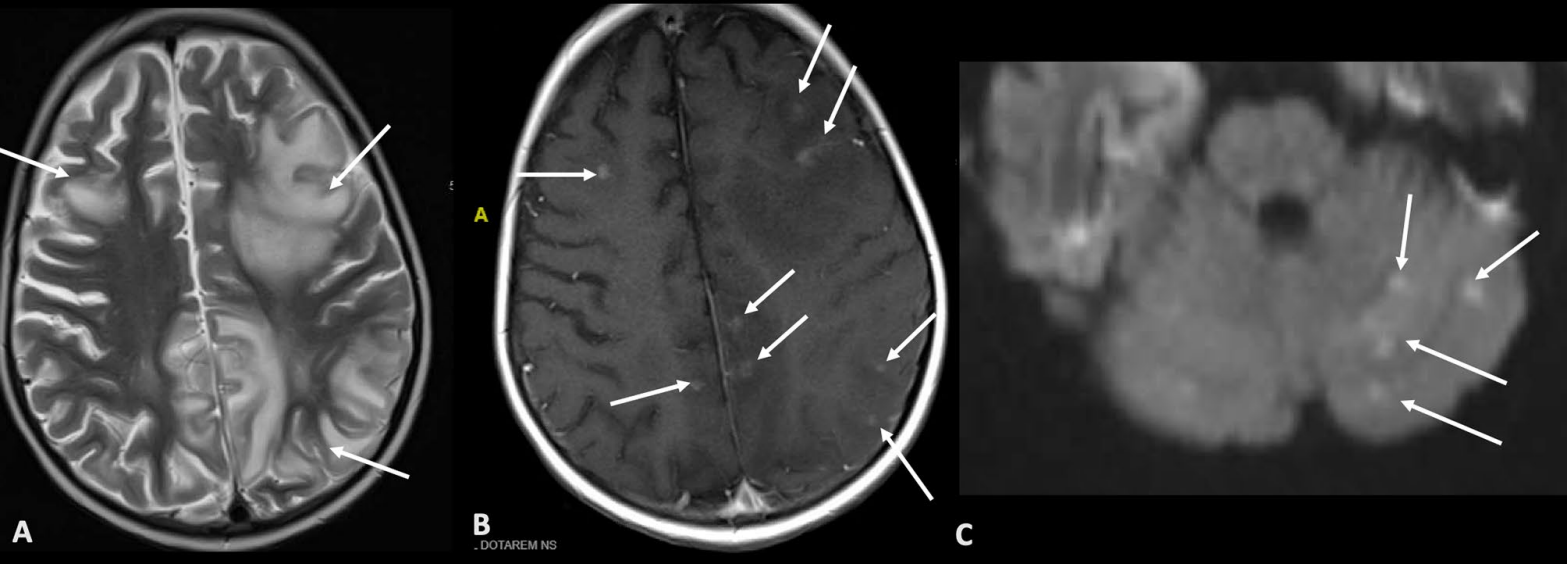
MRI brain images of P6 showing extensive bilateral disease throughout occipital, parietal and frontal lobe cortices. Axial T2 weighted images (A), axial post-contrast T1 weighted images (B) and axial diffusion weighted images (C) show multiple enhancing foci (arrows in B) with prominent surrounding oedema and show showing diffusion restriction (arrows in C). Imaging might suggest fungal infection. MRI – magnetic resonance imaging
